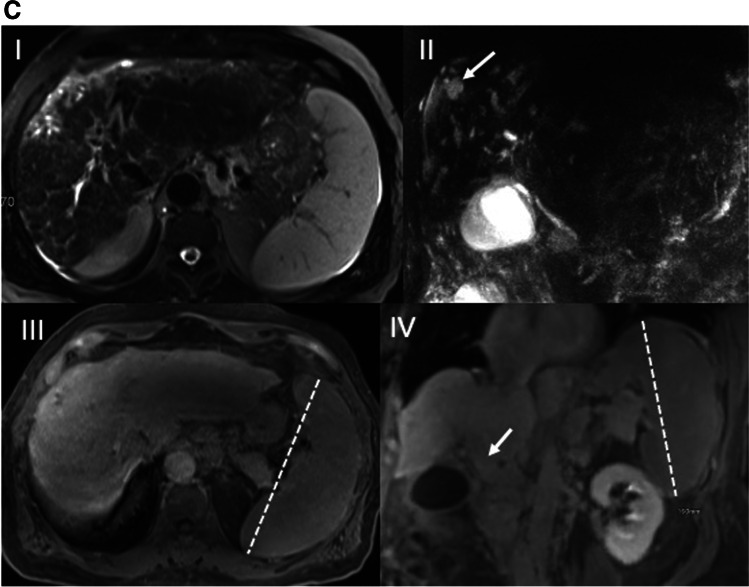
A A 56-year-old male with known PSC for four years. Conventional T2-MRCP (I) is suspicious for a high-grade stricture (HGS) in the hilum (arrow). Furthermore, moderate to significant dilatations and irregularities of the IHD are seen. Axial DWI (II) shows dilatation of the intrahepatic bile ducts in the right liver lobe (short thick arrow), as well as associated high SI in both lobes consistent with segmental cholangitis (thin arrows). Twenty-minute coronal HBP image (III) shows timely contrast media excretion via CBD into the duodenum (arrow), excluding a functional stricture. Thus, this was a false-positive MRCP diagnosis of DS and HGS. The patient developed no adverse event(s). B A 20-year-old female with PSC and a 2-year history of Crohn’s disease. Coronal MRCP (I) shows a suspected significant hilar stricture (arrow) with distinct dilatation of the intrahepatic bile ducts. On the 20-min coronal hepatobiliary phase (II), no contrast media excretion was seen in the biliary system compatible with a functional stricture (FS). There was also heterogeneous liver parenchymal enhancement. ERCP (III) 1 week later confirmed the diagnosis of FS in the mid-CBD, which was treated with dilatation. Three-month follow-up MRCP (IV) showed timely biliary excretion 20 min after contrast administration, as well as more homogeneous liver parenchymal enhancement, indicative of improved function after removal of the FS. C A 62-year-old male with PSC for 23 years. Axial T2-TSE (I), coronal 3D-MRCP (II), and axial (III) and coronal (IV) T1 3D GRE in the HBP show signs of advanced PSC on MRCP with cystic dilatation (II, arrow), and multiple stenoses of the intrahepatic bile ducts, as well as a pruned tree appearance (I, II). The liver shows diminished uptake of hepatobiliary contrast agent relative to the left kidney (III, IV) without excretion at 20 min (IV, arrow), indicative of hepatocellular dysfunction (HD) with signs of cirrhosis, including dysmorphy and portal hypertension (including splenomegaly, III and IV). Four months later, the patient underwent OLT
